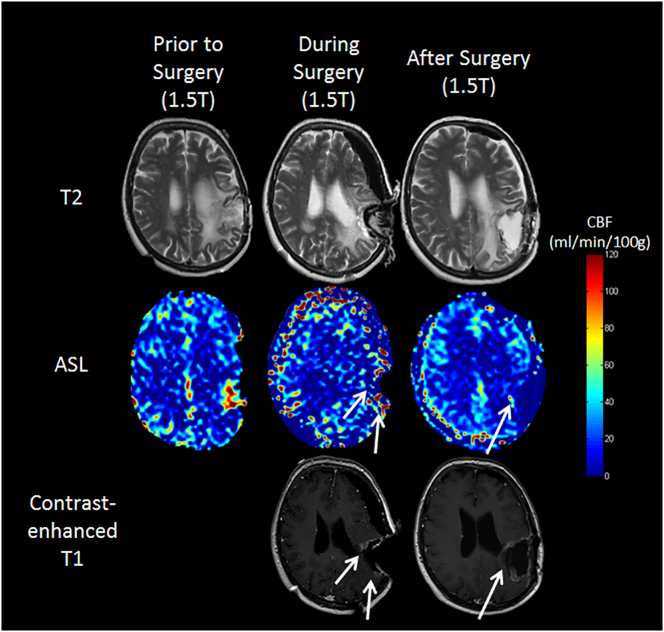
Images of one GBM patient (No. 6), whose tumor could not be resected in total (Table 2). Note that this patient shows rather strong brain shift, making interpretation of both ASL and anatomical imaging more difficult. The patient presented with complete preoperative MR imaging; thus, our data were acquired on 1.5T (pre- and intraoperatively in the OR) and according to the protocol postoperatively. In the preoperative scan, the area of elevated CBF can be clearly seen in the left parietal lobe (left column, middle). In both the ASL and structural imaging, residual tumor mass could be depicted during surgery (middle column, arrows). In the postoperative resection control (right column) the residual tumor mass is only seen as an area of elevated CBF in the ASL scan. On the contrast-enhanced T1-weighted images the residual tumor is not as prominent as any surgically induced changes and was overlooked at first in the blinded review.
